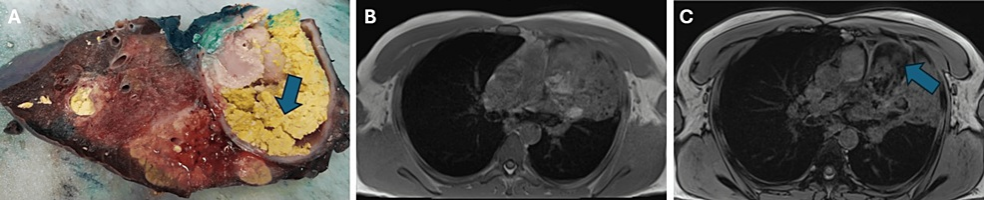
Macroscopic appearance and magnetic resonance imaging (MRI) of the mediastinal teratoma. Teratoma is represented in image (A) (obtained from the Department of Pathology), which displays cysts and sebaceous material components (arrow). Axial T1 in-phase (B) and opposed-phase images (C) showing hypointensity areas in T1 opposed-phase image in the sebaceous material (arrow).
